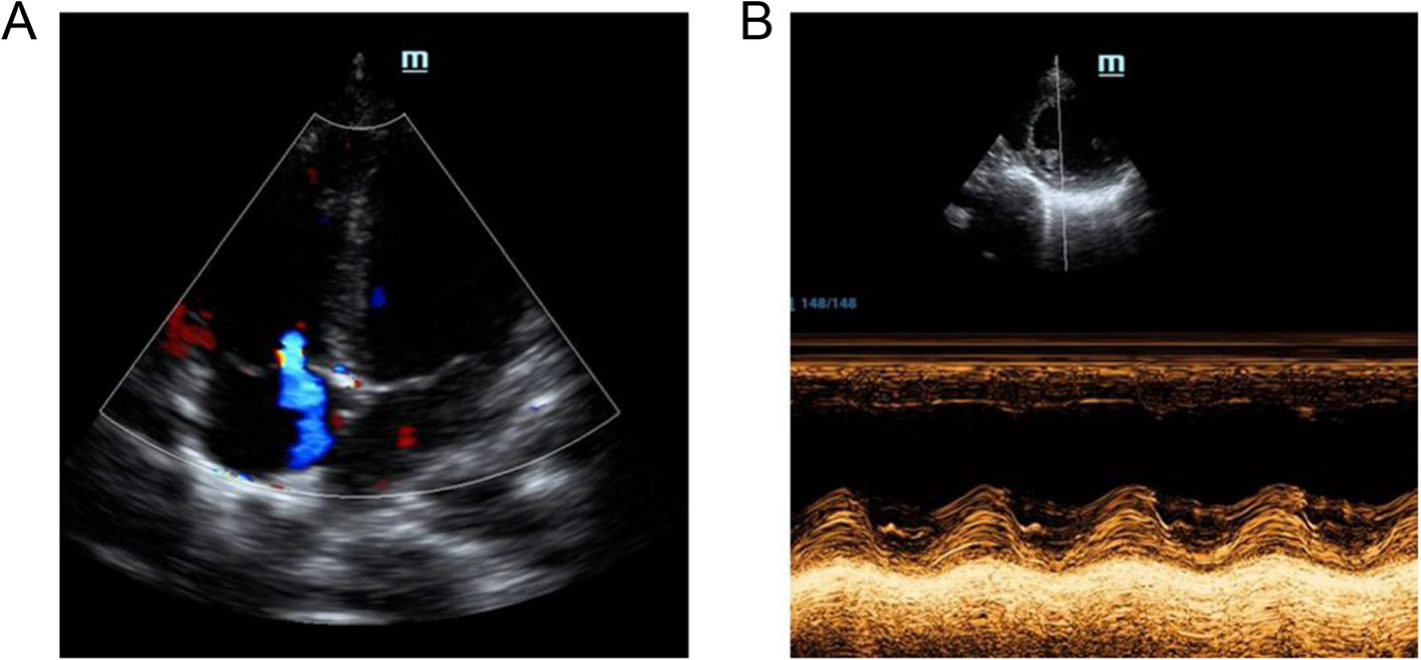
a In transthoracic echocardiography, local anterior wall dysfunction has been observed. b M-mode echocardiography showed left ventricle ejection fraction was good
